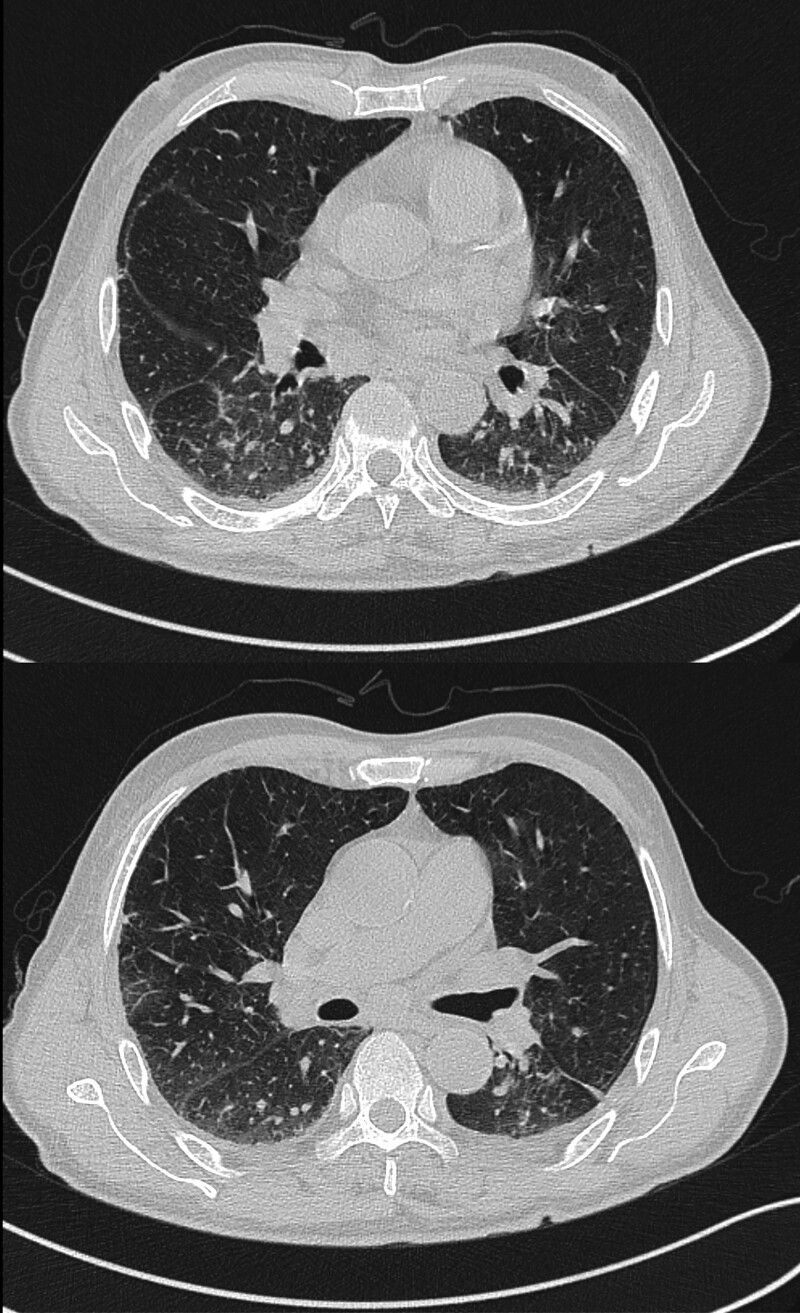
Chest computed tomographic (CT) scan. Showing multiple enlarged lymph nodes, interstitial changes with inflammation and small inflammatory nodules in both lungs.
